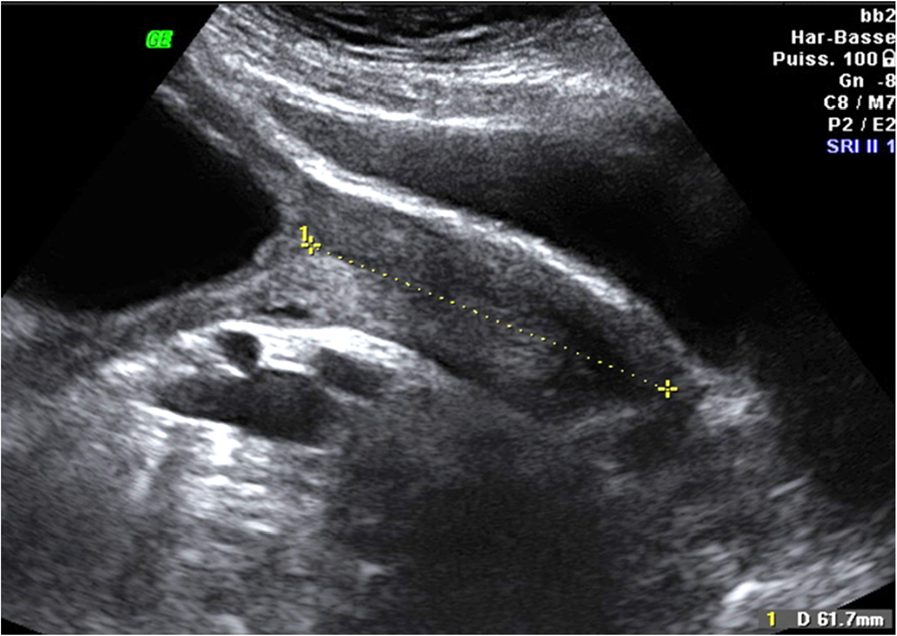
Sonographic measurement of cervical length in a woman with achondroplasia (16 weeks of gestation). Cervical length measurement using abdominal sonography of a woman with achondroplasia (16 weeks of gestation)
